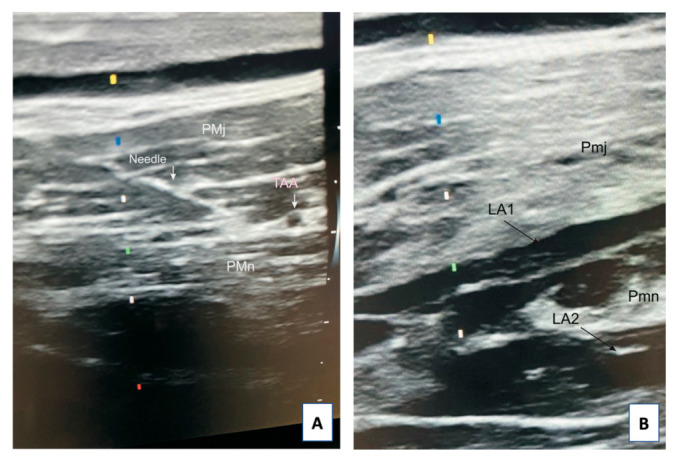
Sonogram of the needle position during the application of the modified PECS II (A) and local anesthetic deposition after application of the modified PECS II block (B). Abbreviations: Pmj, pectoralis major muscle, Pmn, pectoralis minor muscle, TAA, thoracoacromial artery, LA1, local anesthetic spread into the fascial plane between Pmj and Pmn, LA2, local anesthetic spread into the fascial plane underneath Pmn.
